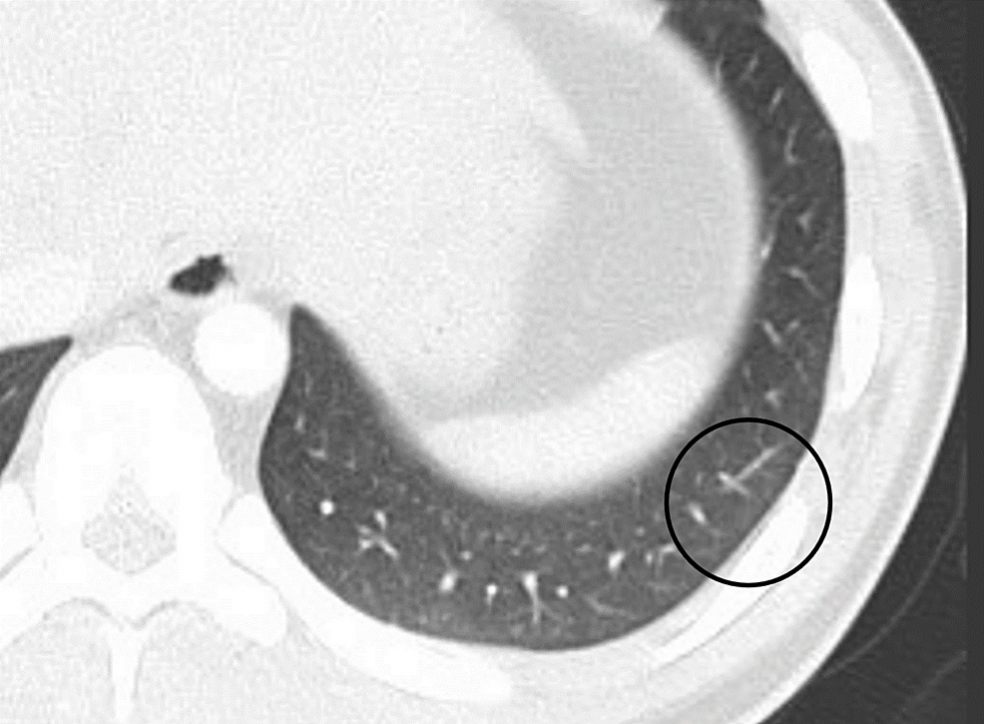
CT scan was performed again, and the frosted shadow of the lower lobe of the left lung had disappeared. CT: computed tomography
